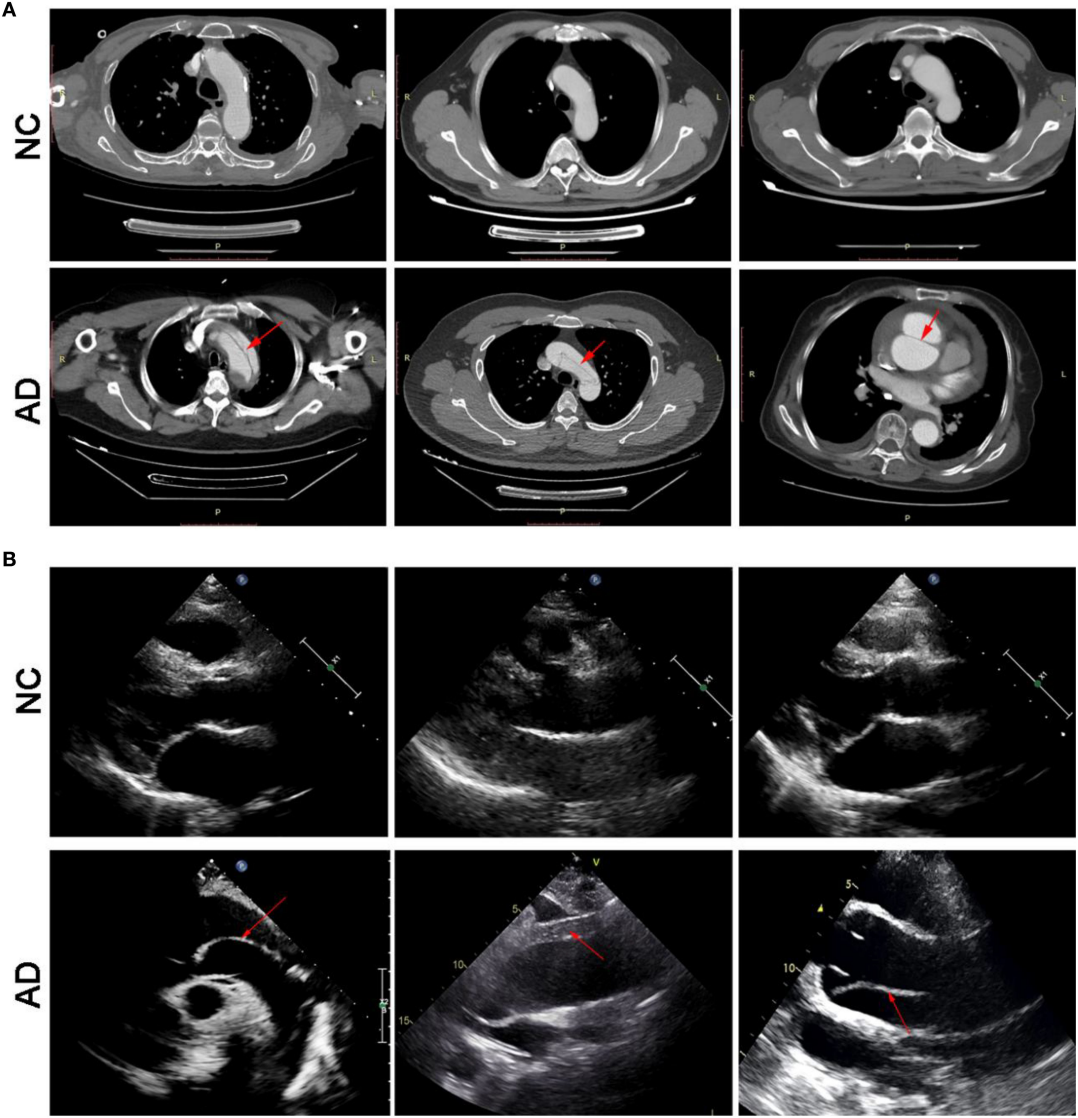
Transverse view of aortic computed tomography angiography (A), and transthoracic echocardiography (B).
